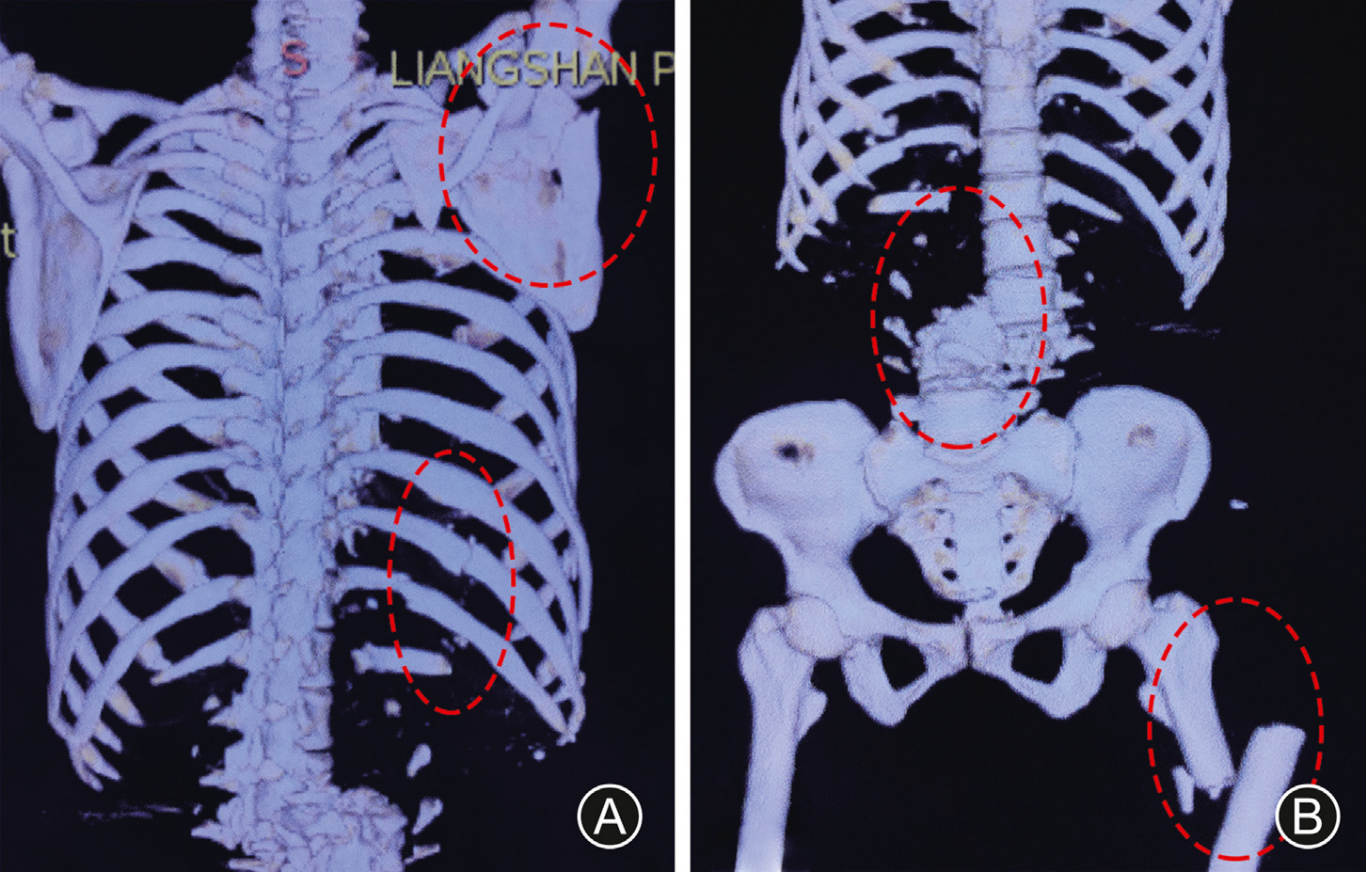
Three-dimensional reconstruction of CT scans in local hospital. Scapular fractures and rib fractures (A), L_3_ spondyloptosis combined with proximal femoral fracture and lumbar vertebra fracture (B) were enclosed by the red ellipse.
